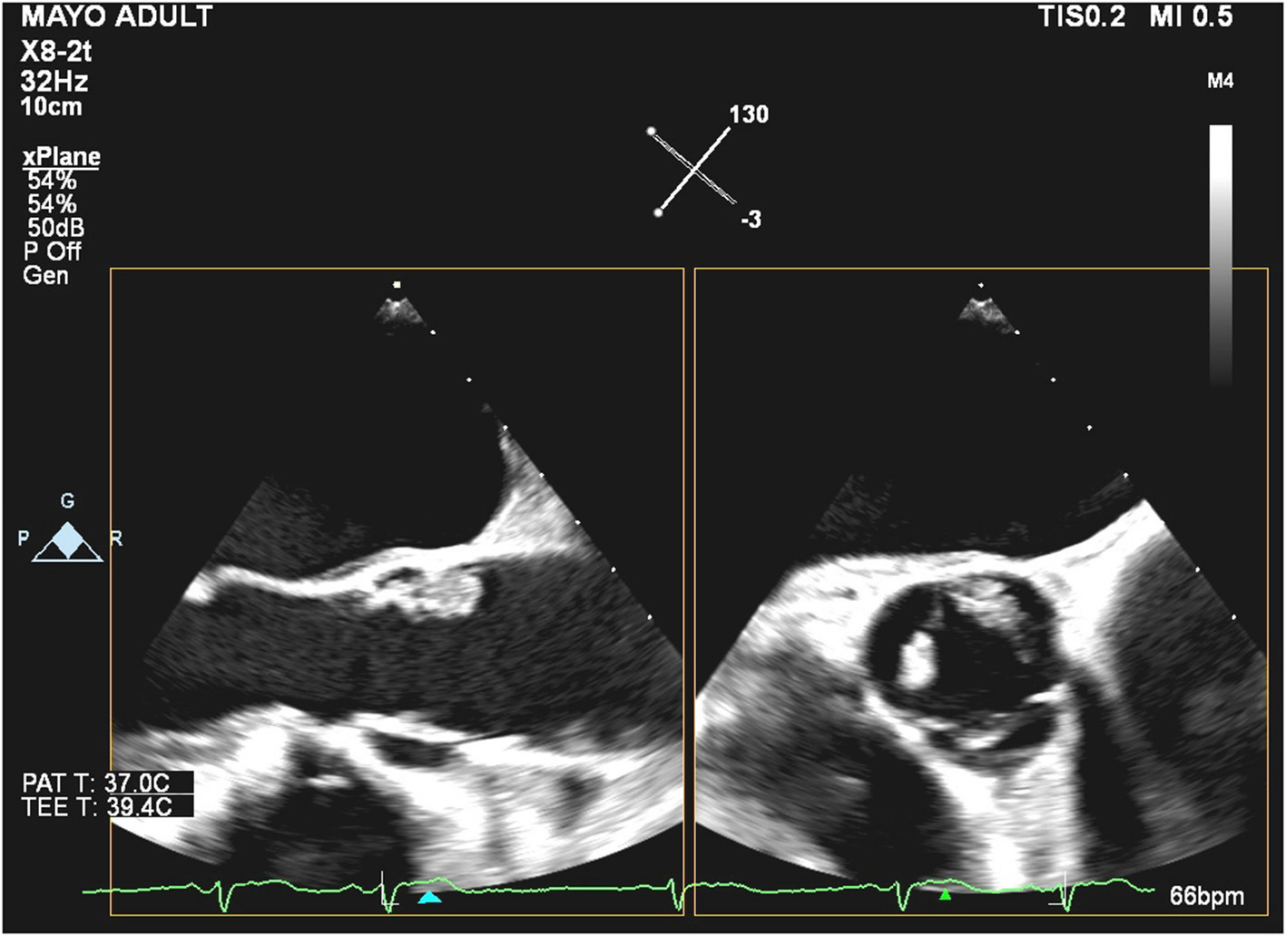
Biplanar transesophageal echocardiography of the aortic valve during systole with the mid-esophageal aortic valve long axis (left) and short-axis (right).
